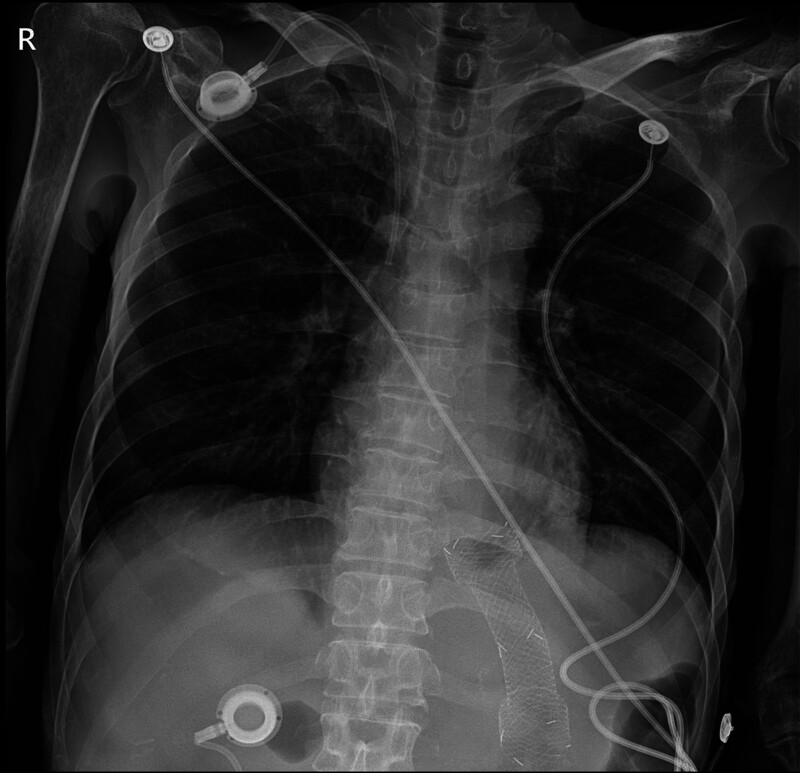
Chest radiography revealing no definitive evidence of pneumopericardium.
